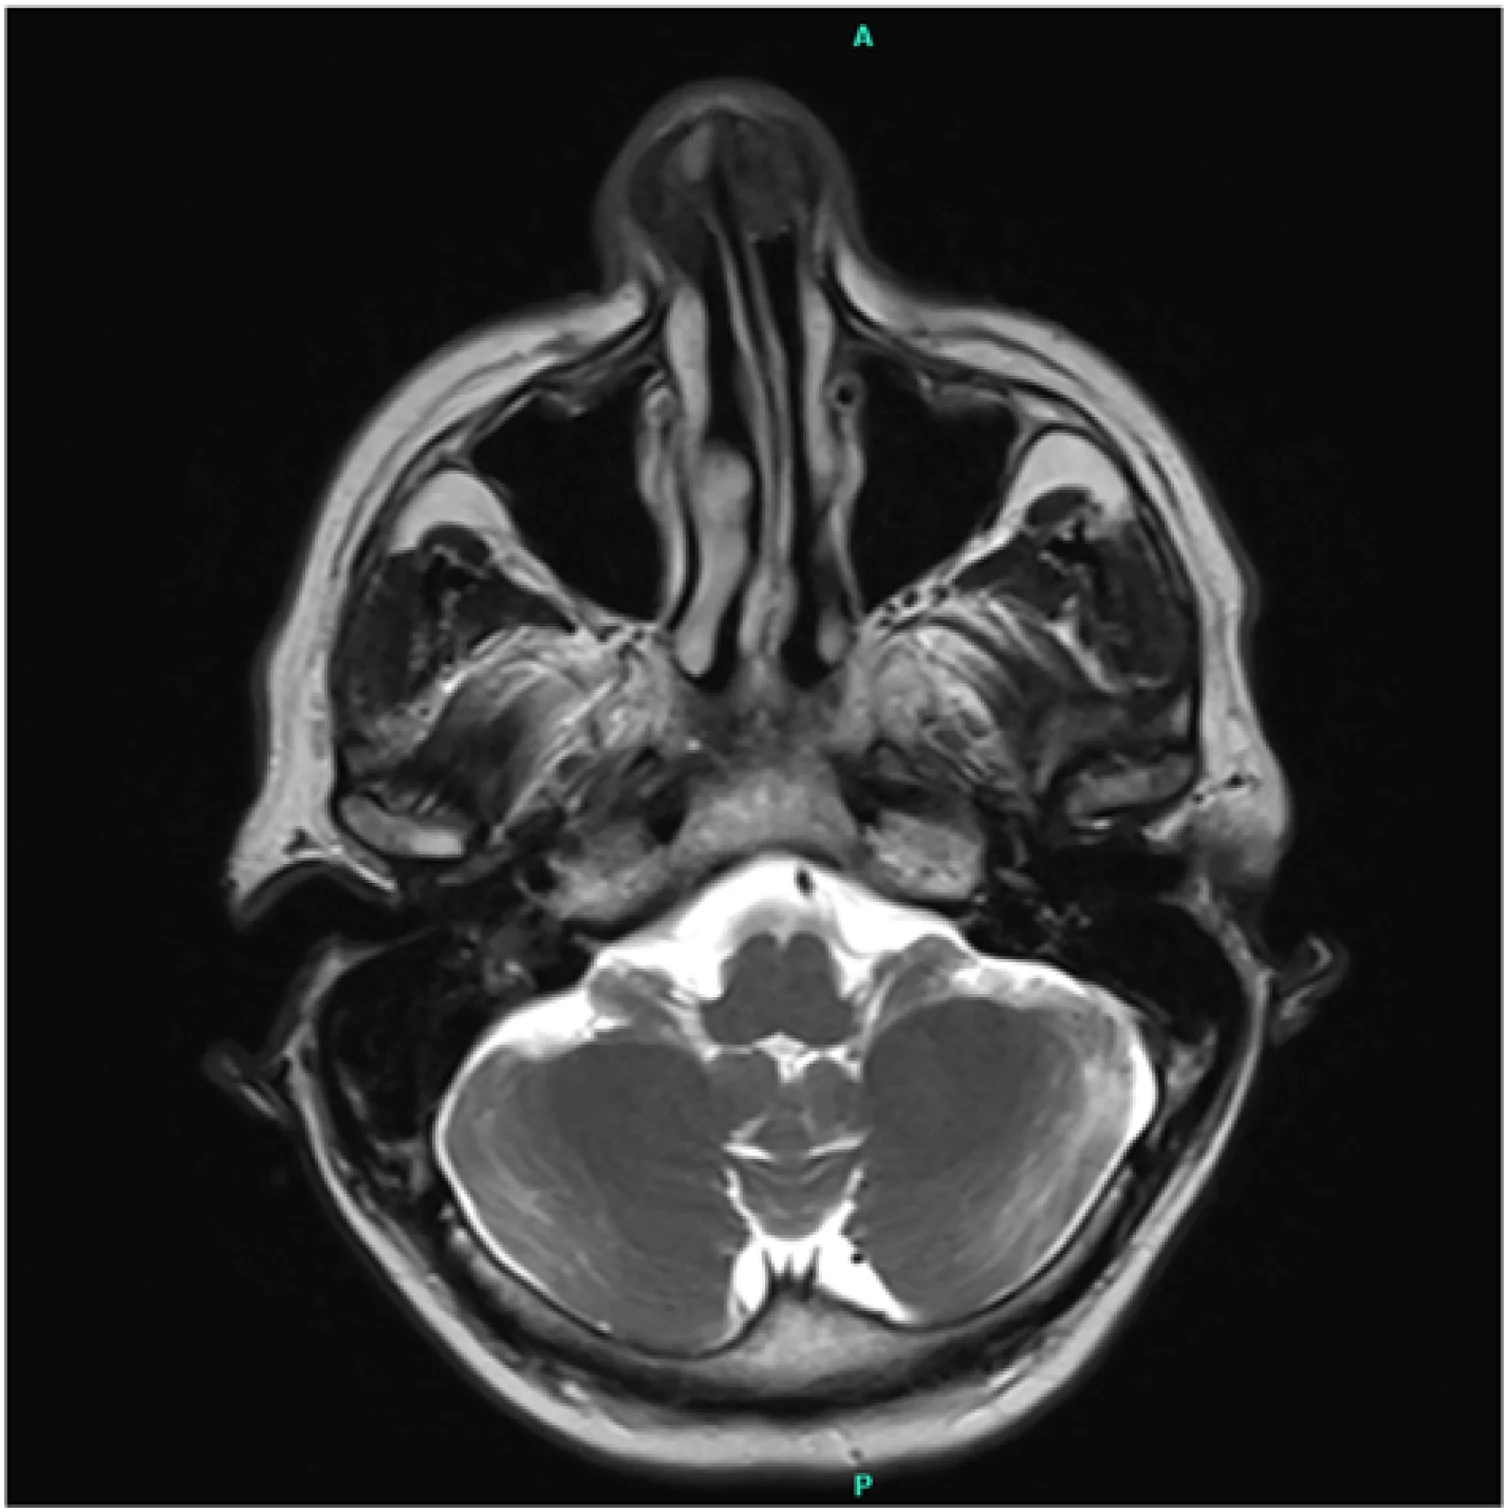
Axial T2-weighted MRI demonstrating a heterogeneous anterior nasal cavity lesion.
